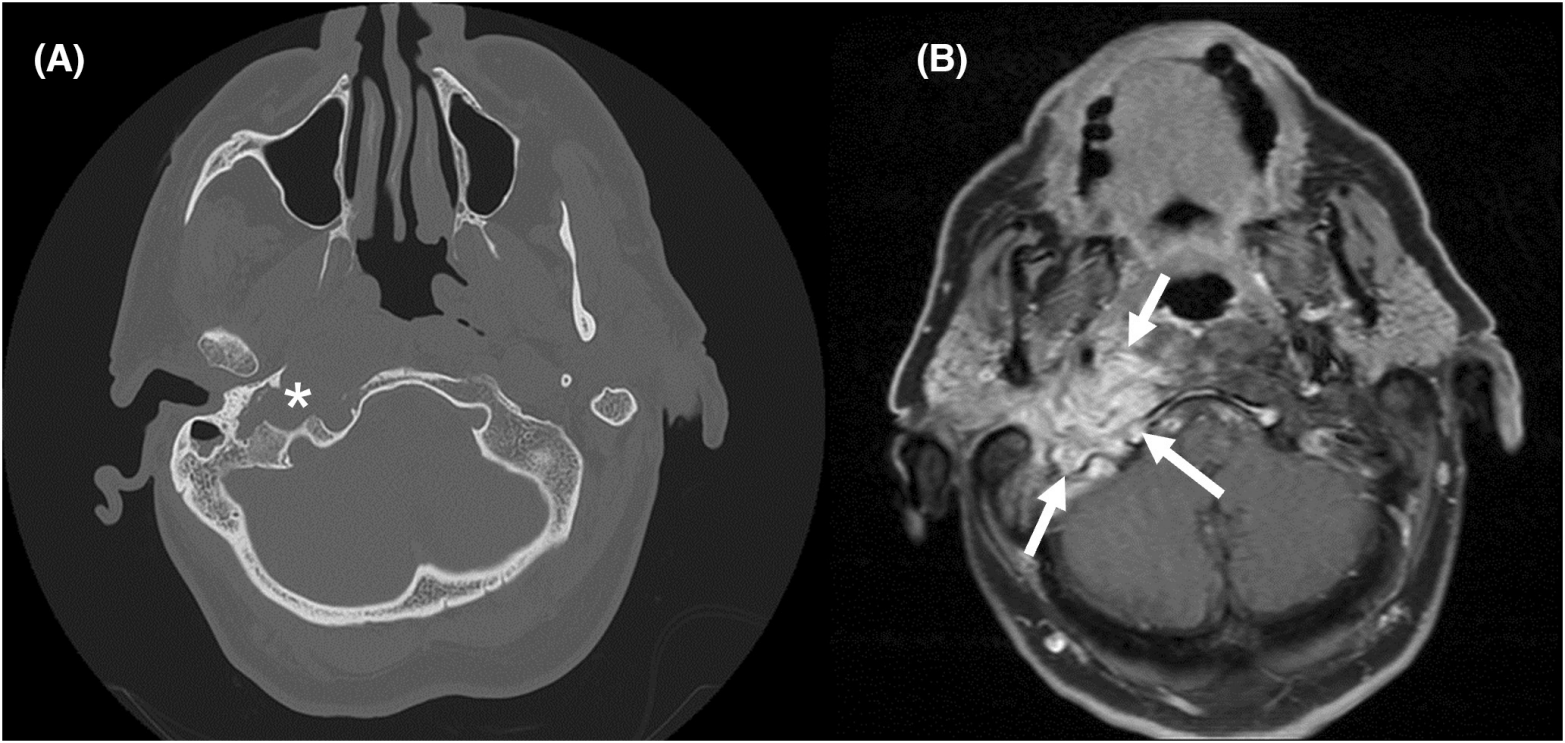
Axial CT (A) and T1 Dixon MRI (B) demonstrating right central skull base bone erosion (asterisk) with infiltrating soft tissue mass (arrows).
